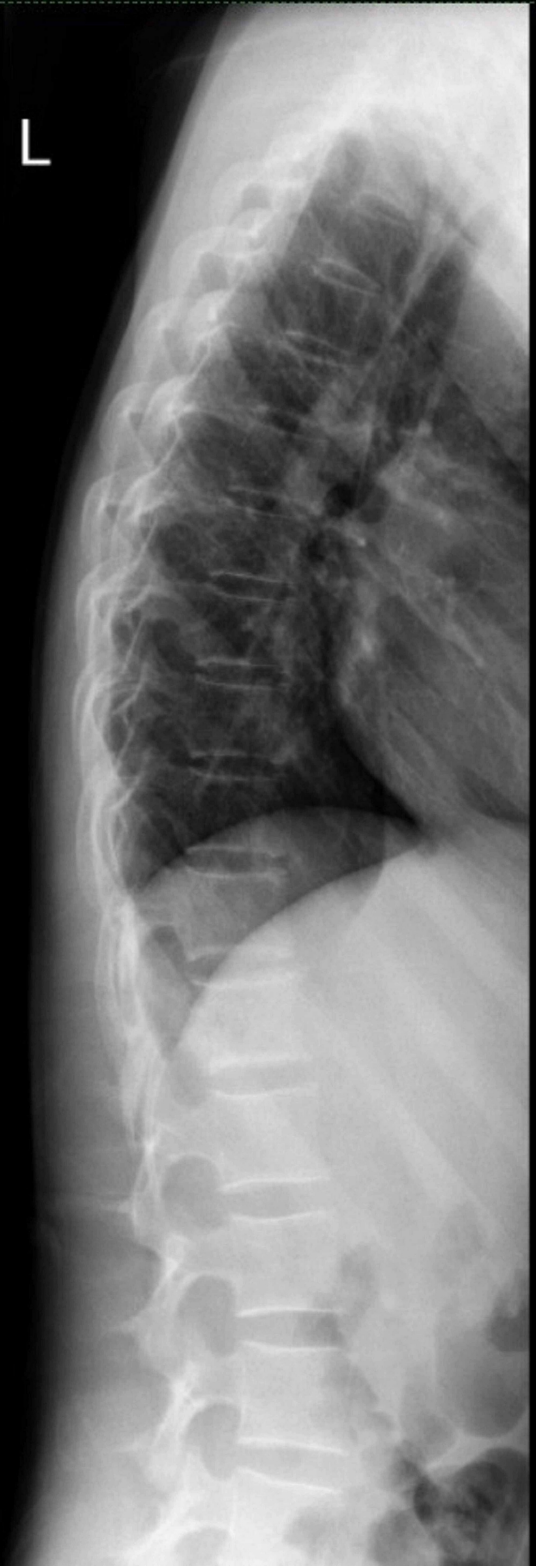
Pre-operative plain radiograph of spine (lateral view).
